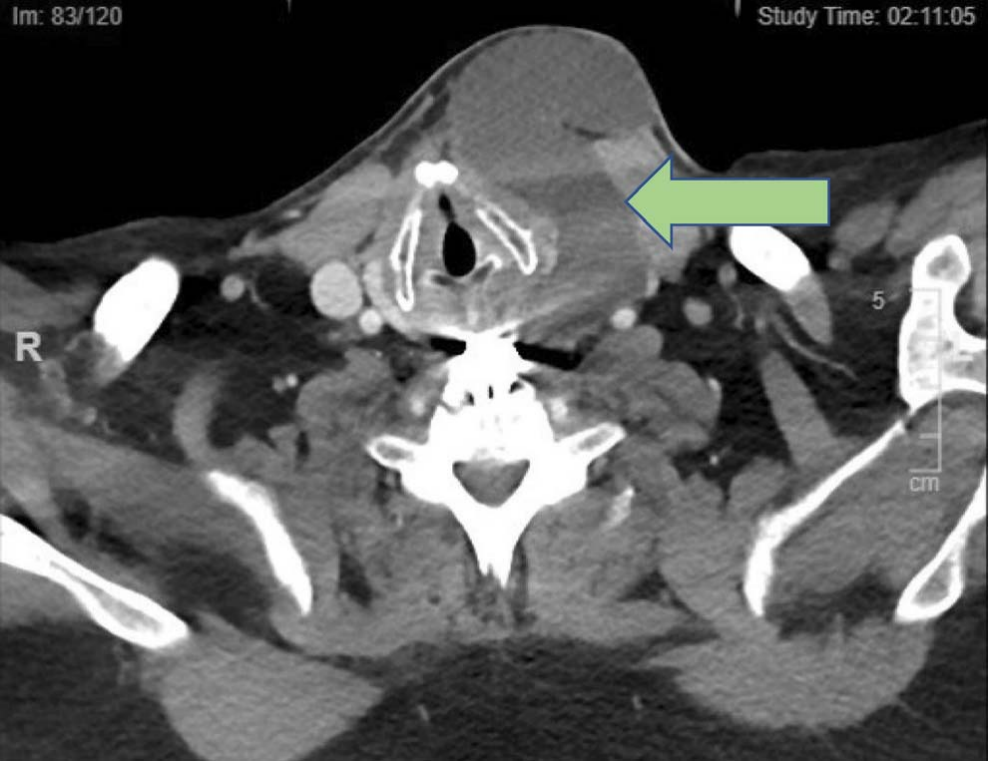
Axial computed tomography image of the neck. Green arrow indicates large cerebrospinal fluid collection emerging from the retropharyngeal space extending to the left of the cricoid cartilage and displacing it rightward, with resulting large anterior mass effect and superior tracheal compromise.
